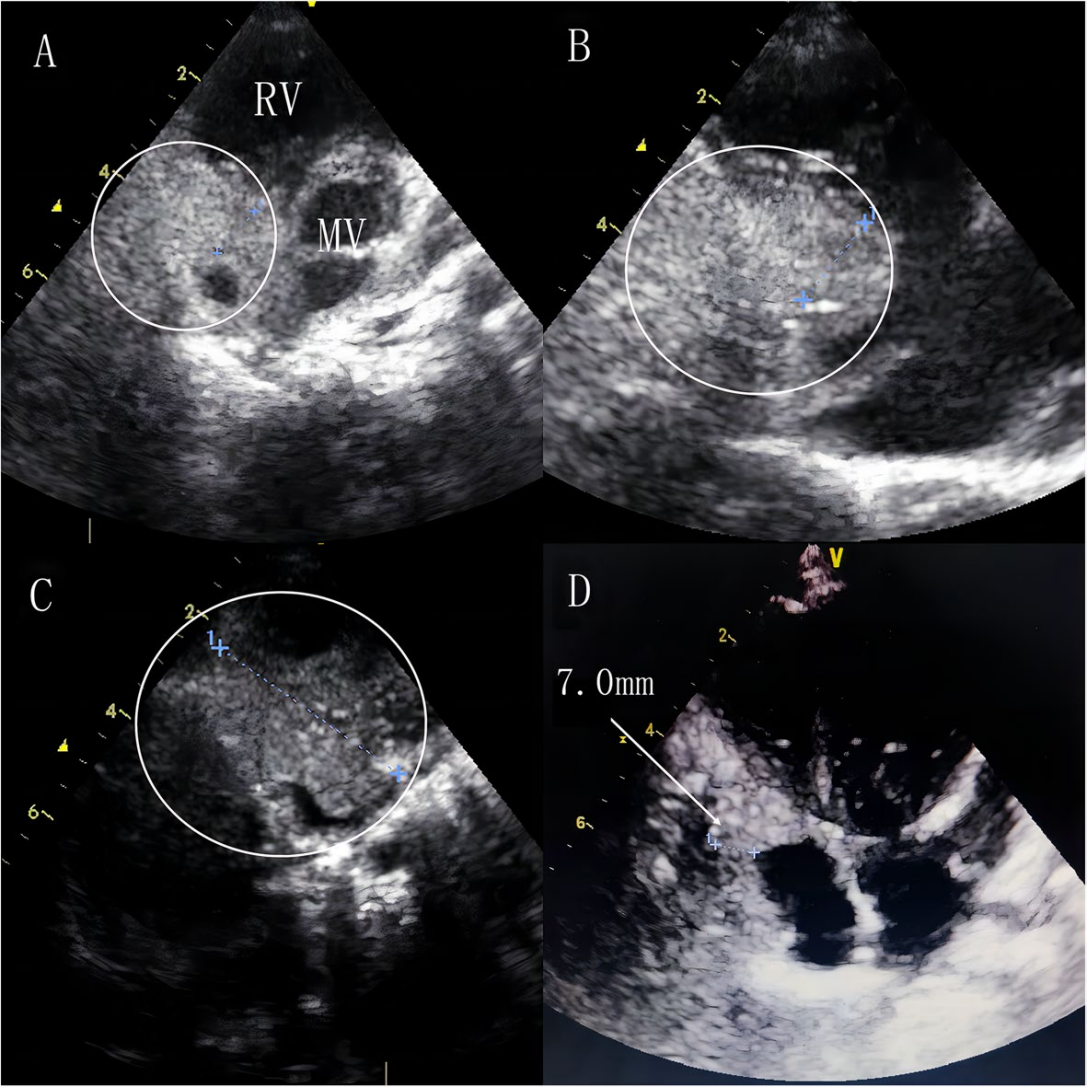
A Transthoracic echocardiogram obtained in the parasternal non-standard four-chamber view showing the mass with uneven echo. B Transthoracic echocardiogram obtained in the right ventricular inflow tract view showing the mass and the atrial septal defect (white arrow). C Transthoracic echocardiogram obtained in the Subxiphoid view showing the mass. D, E Transthoracic echocardiogram showing the mass that has developed two years after the surgery. MV, mitral valve; RV, right ventricle
